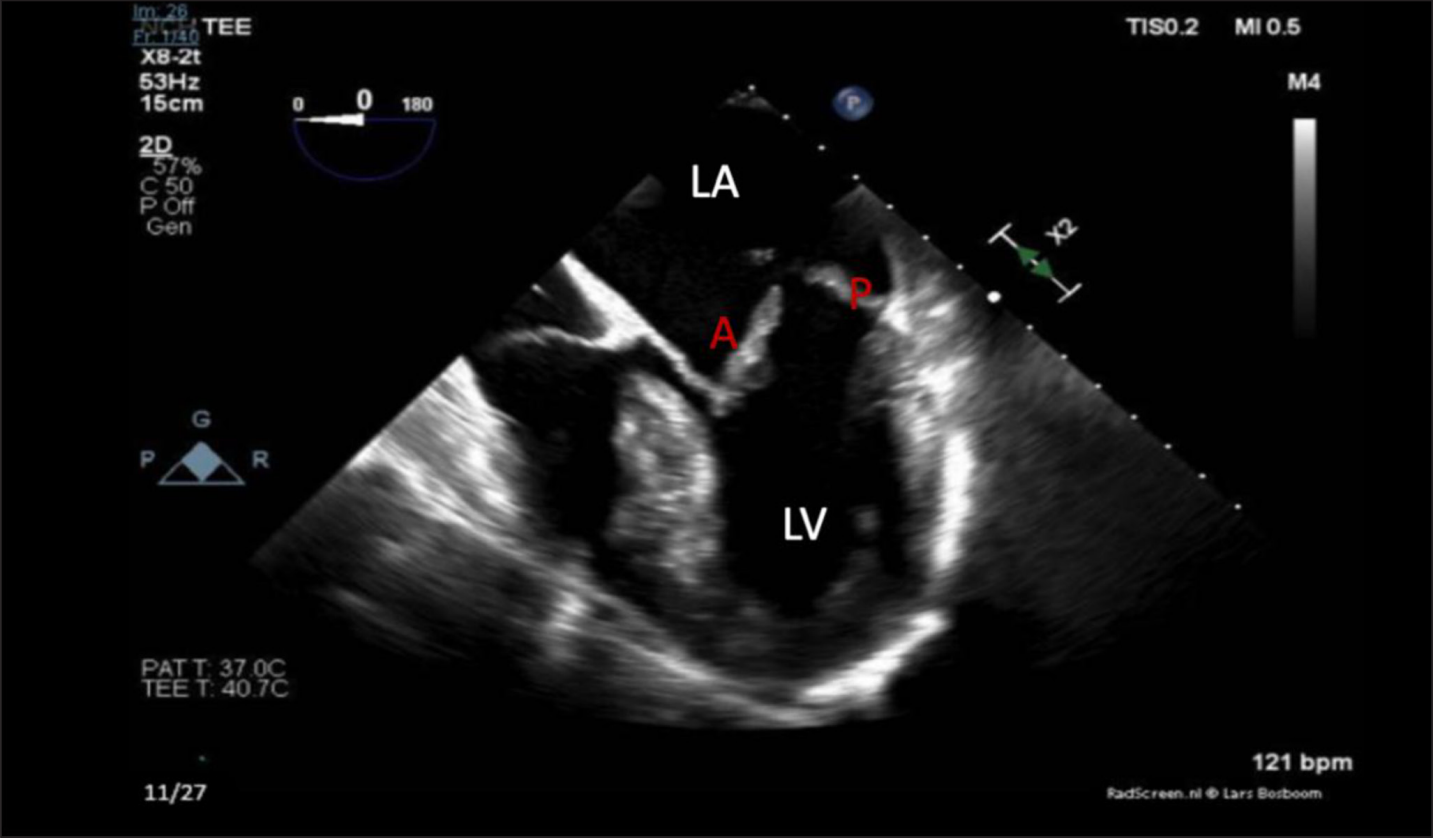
Mid-esophageal transesophageal echocardiogram with 4-chamber apical view view showing left ventricle (LV) and left atrium (LA). Myxomatous degeneration and severe prolapse of the anterior and posterior leaflets of the mitral valve are seen on this image.
